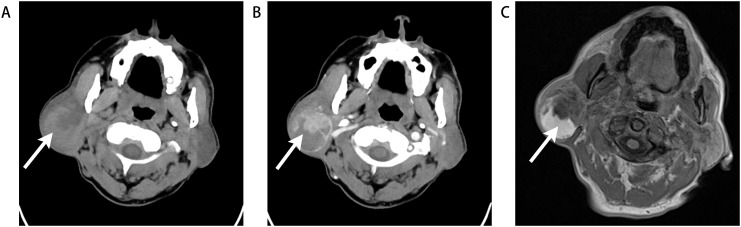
(A) Non-contrast neck computed tomography (CT) imaging shows a round, mixed-density mass (arrow) in the superficial lobe of the right parotid gland, containing hyperdense hemorrhagic foci and relatively hypodense solid components. (B) Contrast-enhanced neck CT demonstrates marked enhancement of the solid components, internal septations and a capsule, multiple small vessels surrounding and within the mass, and non-enhancing hemorrhagic/necrotic/cystic areas. (C) Non-contrast neck magnetic resonance imaging (MRI), axial T1-weighted images reveals a heterogeneous-signal mass in the right parotid gland, with solid components showing iso-to slightly hypointense signals and hemorrhagic/necrotic/cystic areas appearing hyperintense.
